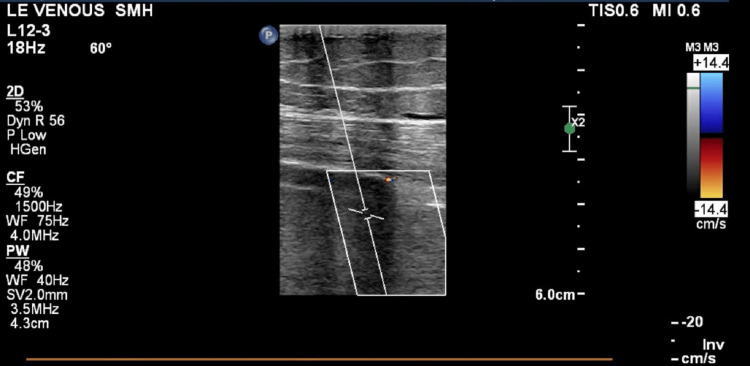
Left leg Duplex venous ultrasound exhibiting no venous flow
